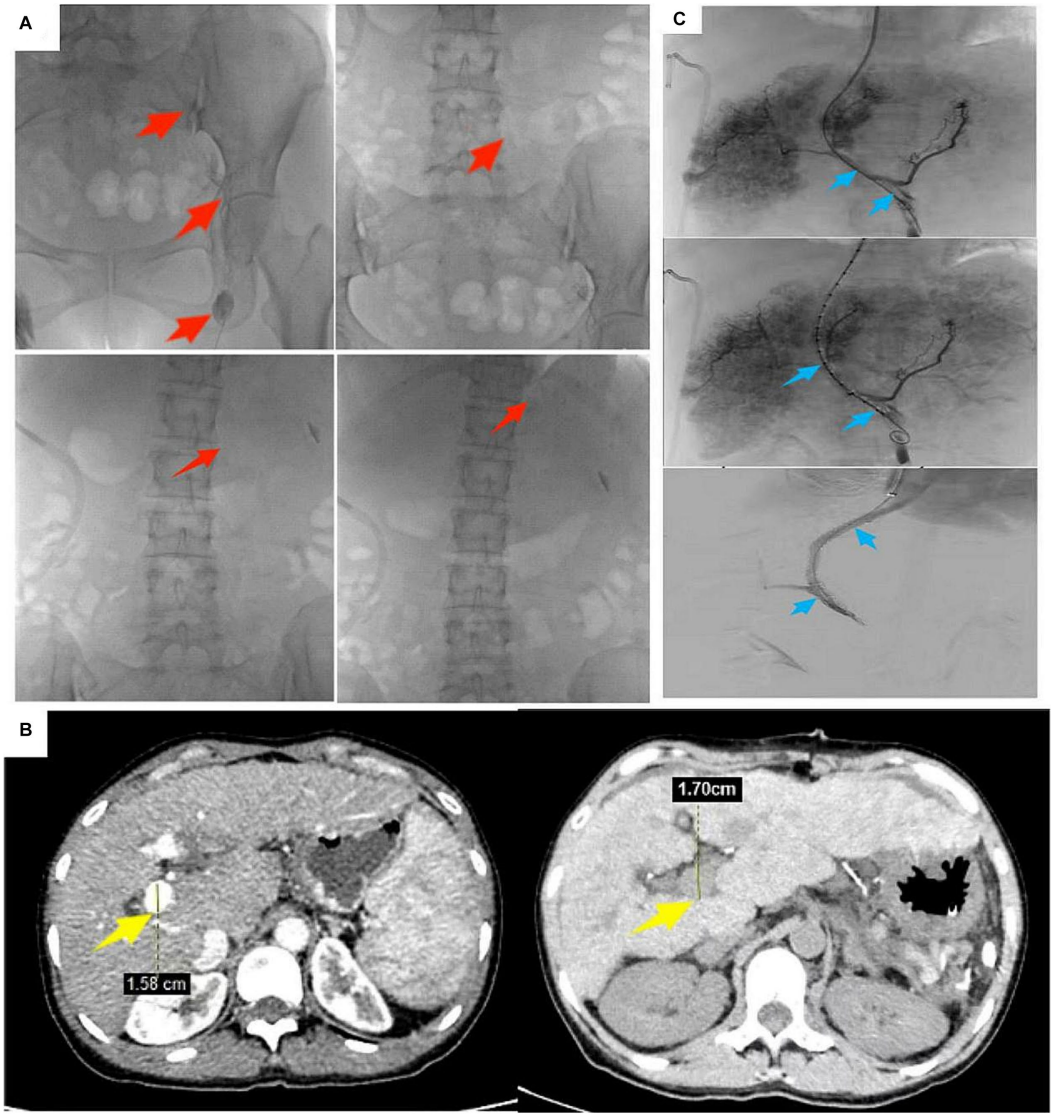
A 47-year-old female patient underwent robot-assisted LSPD. (A) 165 days after LSPD, ultrasound-guided lymphangiography through the inguinal region showed no abnormalities in the shape of the pelvic and lumbar lymphatic vessels, and no visualization of the thoracic lymphatic vessels (red arrows); (B) Compared with the pre-LSPD, the portal vein width before TIPS increased from 1.58 cm to 1.7 cm (yellow arrow); (C) During TIPS treatment, thrombosis in the portal vein was found. The patient underwent TIPS treatment under general anesthesia 169 days after LSPD (blue arrow).
